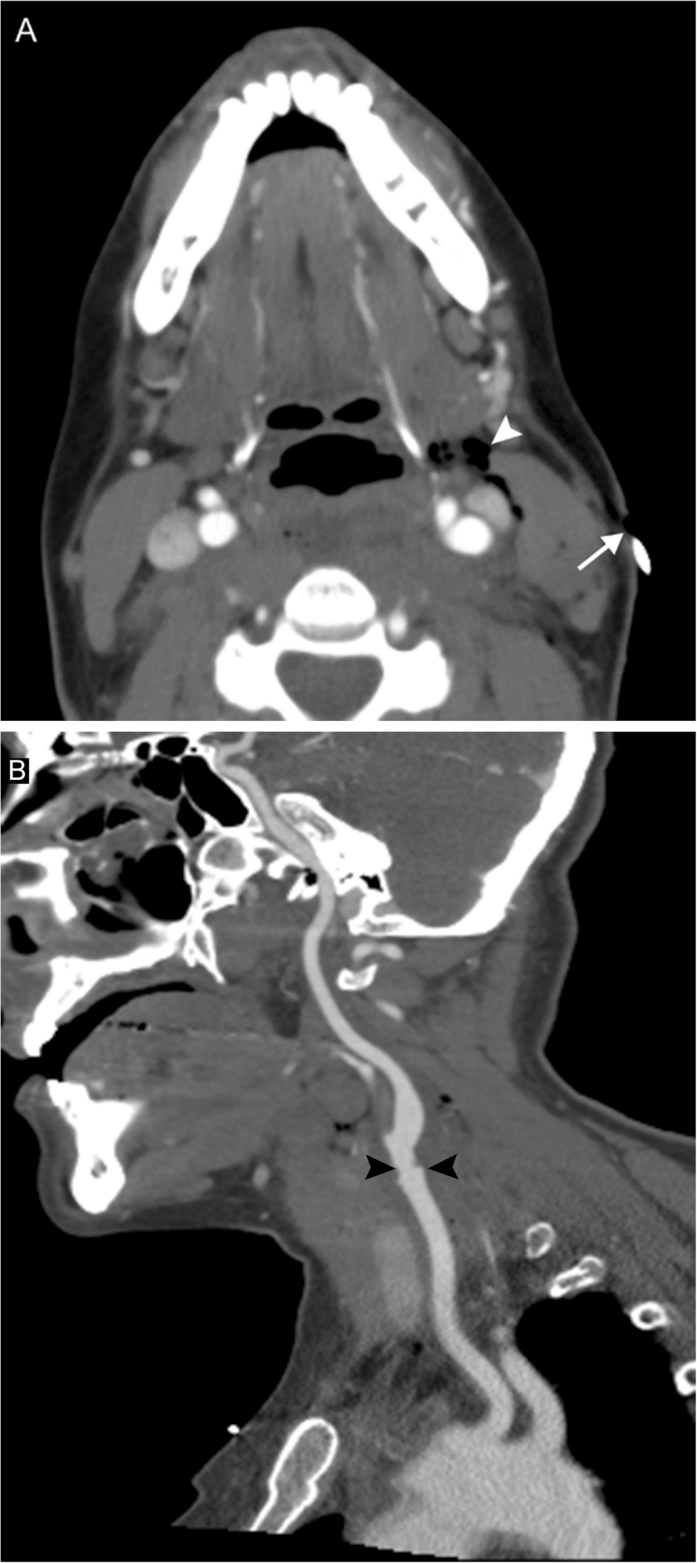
Carotid space trauma. A 33-year-old woman who sustained a stab wound to the neck, subsequently noted to have a weak voice; laryngoscopy confirmed vocal cord paralysis. Axial CTA of the neck (a) reveals a radiopaque marker at the entry site (white arrow); air is seen within the deep soft tissues anterior to the left carotid sheath contents (white arrowhead), indicating violation of the left carotid space. 3D-volume-rendered CTA image (b) of the left carotid artery shows irregular caliber and focal outpouching of the distal left common carotid artery (black arrowheads), representing pseudoaneurysm. Findings are consistent with left vocal cord paralysis due to vagal nerve injury
